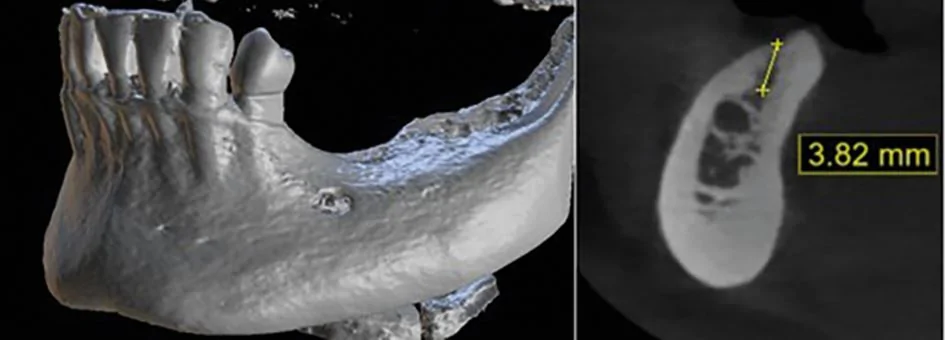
Cone beam computed tomography render of the pre-operative situation. Mental foramen just posterior to the lower left first premolar
